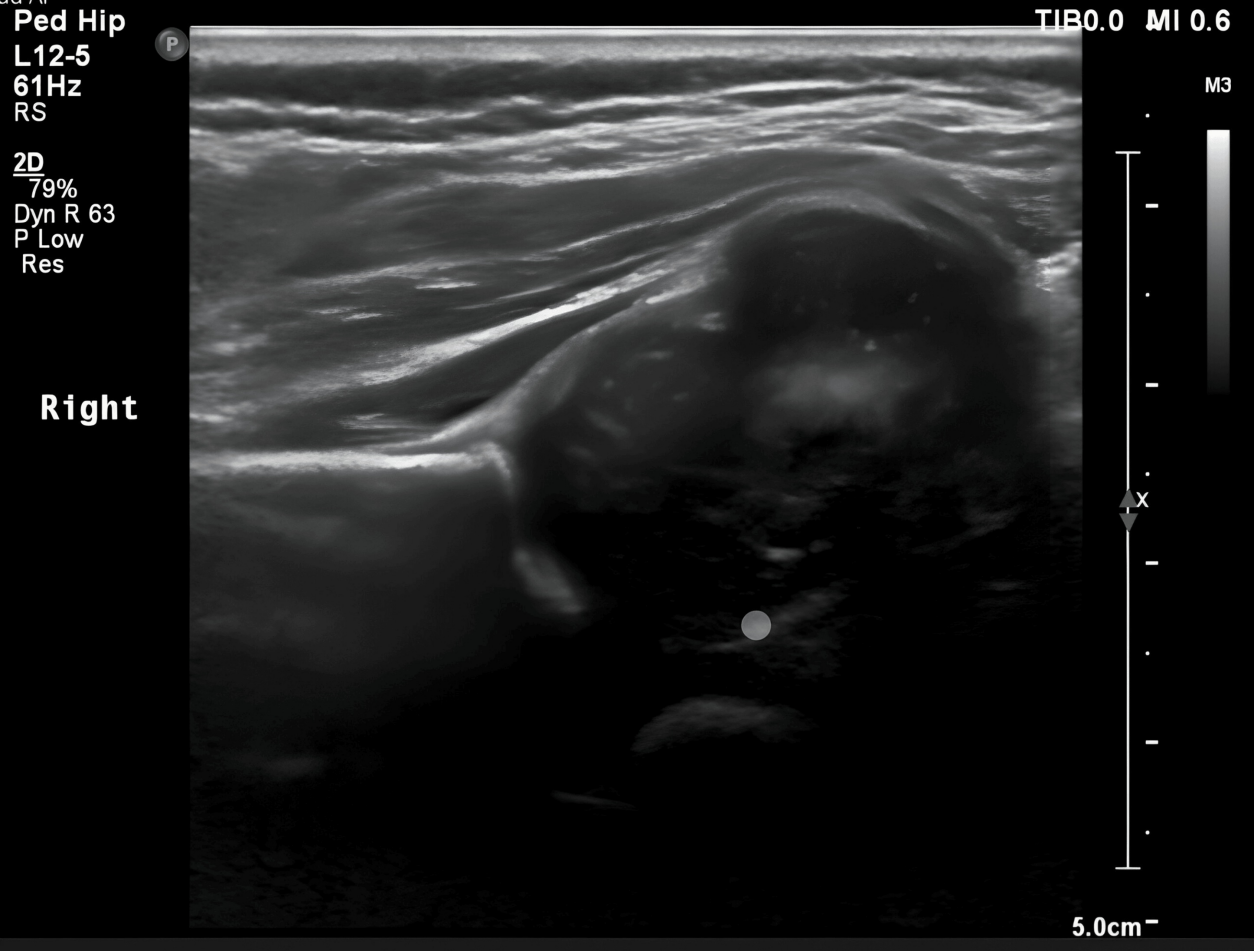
Ultrasound that identifies the collection
